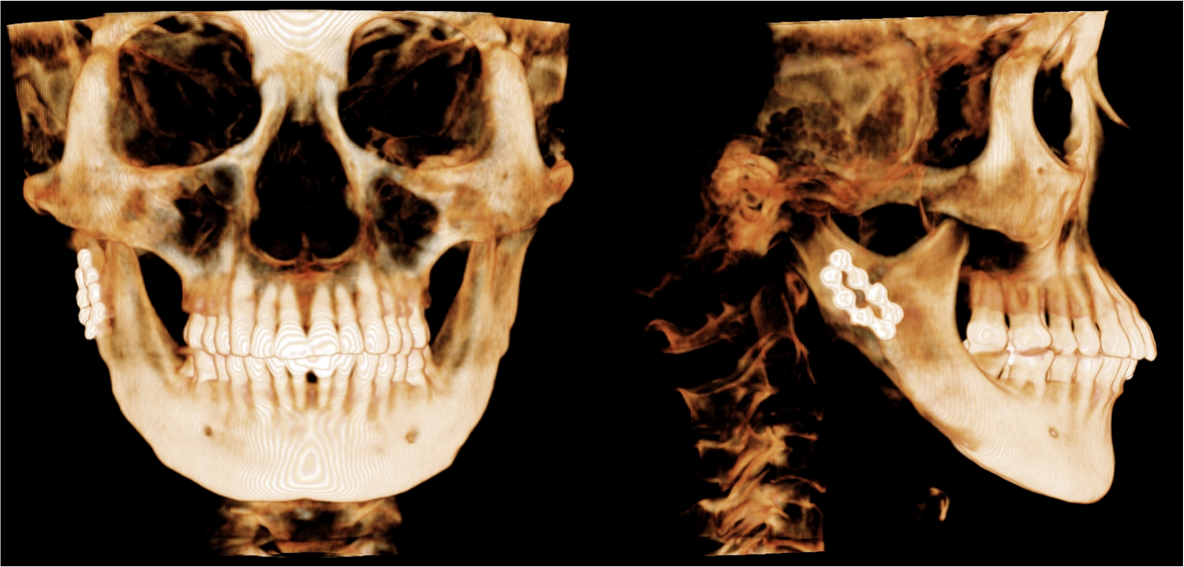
Postoperative 8-month 3D-CBCT, frontal and right lateral view, showing no distinct displacement of condylar fragment
